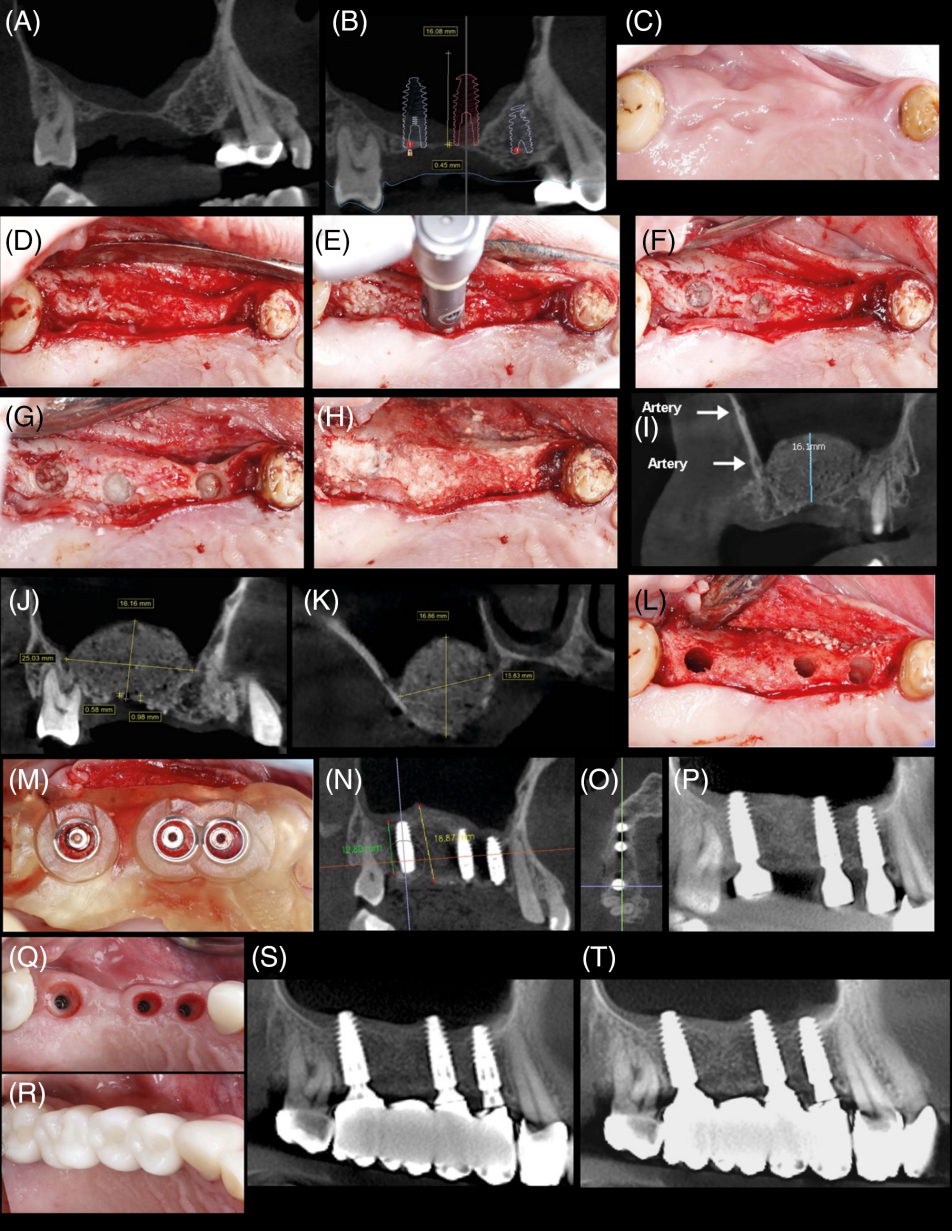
(A–T) Advanced sinus grafting with osseodensification 2‐stage Protocol III in right maxillary quadrant (courtesy Dr. Marcel Firlej). Combined crestal sinus grafting with localized lateral ridge expansion of severally resorbed alveolar ridge with second‐stage approach post augmentation for implants placement: patient presented with missing maxillary right first and second premolar, and first and second molar with severe crestal alveolar bone height deficiency and localized horizontal alveolar ridge resorption in the right first premolar site. Crestal bone height ranged from <1 mm in molars sites to ≥5 mm in premolar area. (A) Initial CBCT scan of the maxillary right quadrant; (B) digital treatment plan; (C) initial clinical occlusal view of the maxillary right edentulous area. (D) Occlusal clinical view with flap reflection showing localized narrow alveolar ridge in the first and second premolar sites. (E,F) OD bur VT4555 (5.0) was used in osseodensification mode (1000 RPM CCW with irrigation) with vertical G‐stop to enter the sinus in molars sites to facilitate initial Schneiderian membrane separation off the sinus bony bed with autogenous bone compaction grafting into the sinus. Note molar sites osteotomies with intact Schneiderian membrane. (G) Occlusal clinical view of the final osteotomy created with particulate allograft in the molar area grafting the sinus, allograft was slowly compacted with OD bur VT4555 (5.0) running in 100 RPM to achieve adequate sinus grafting. Ridge expansion was done in the premolar site using OD burs according to osseodensification ridge expansion protocol. (H) Occlusal clinical view of the final three osteotomies fully grafted with cancellous/cortical allograft. (I) CBCT scans for verification in the day of sinus augmentation surgery with cancellous/cortical allograft. Note the extent of the membrane lift to 16 mm. (J) 4 months post‐surgery follow‐up CBCT confirming adequate healing of the augmented upper right sinus and (K) is after 8 months post‐surgery and prior to implant placement. (L,M) Occlusal clinical view of implants osteotomies and placement in area of first premolar, first molar, and second molar. Densifying burs were used in osseodensification mode (1000 RPM CCW) to create the osteotomies through the surgical C‐guide (Versah, LLC). (N,O) CBCT scans of the implant's placements (8 months post initial sinus grafting / ridge expansion). Note: adequate vertical and horizonal upper right alveolar ridge augmentation with sinus grafting. (P) CBCT scan at day of implant uncover at 4 months post implant placement. (Q,R) Occlusal views and (S) CBCT scan of healing at 6‐months post implant placement with implants supporting a fixed dental prosthesis. (T) 3‐years follow‐up of CBCT scan demonstrating a stable osseointegration of the three implants placed in the grafted sinus with maintained crestal bone and sinus graft height.
